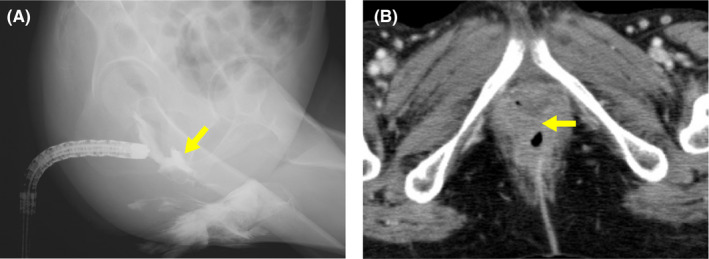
Radiological findings suggestive of rectovaginal fistula observed at 9 months from initiation of radiotherapy. (A) Barium enema radiography. The arrow indicates leakage of contrast enhancement agents (injected into the rectum) into the vagina. (B) Computed tomography. The arrow indicates the rectovaginal fistula
